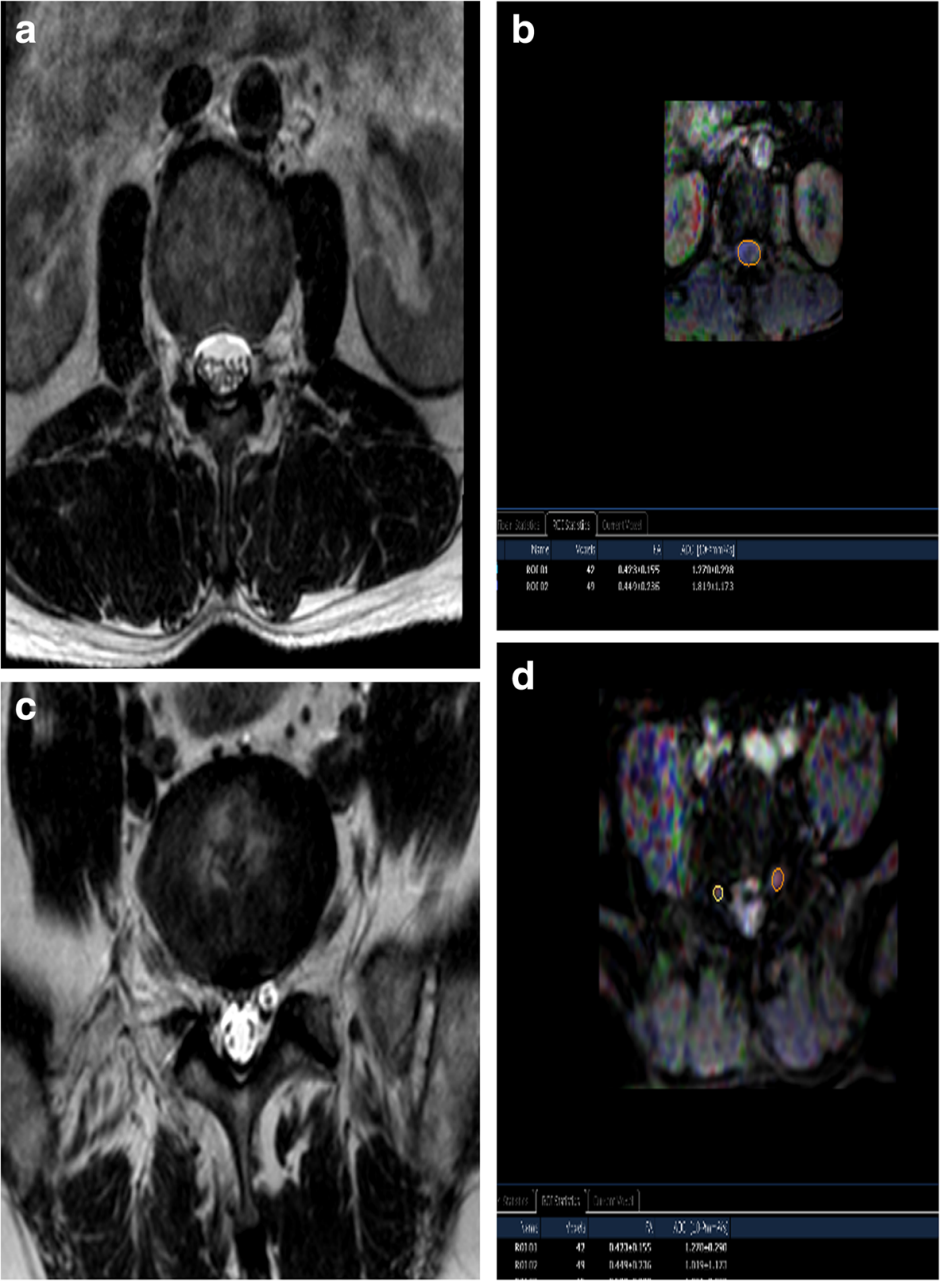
MRI T2W image of cauda equina (a) and FA mapping of DTI of cauda equina (b). ROIs were placed on the cauda equina on the zones equally as the disc, including superior 1/3, middle 1/3, and inferior 1/3 of the disc on FA mapping and FA values were calculated (b). The minimum values of three zones were taken as FA values of the cauda equina; MRI T2W image of bilateral nerve roots (c) and FA mapping of DTI of bilateral nerve roots (d). ROIs were placed on the “intraspinal,” “intraforaminal,” and “extraforaminal” zones of bilateral nerve roots on FA mapping and FA values were calculated (d). The minimum values of three zones were taken as FA values of the nerve roots. *MRI* magnetic resonance imaging, *FA* fractional anisotropy, *DTI* diffusion tensor imaging
